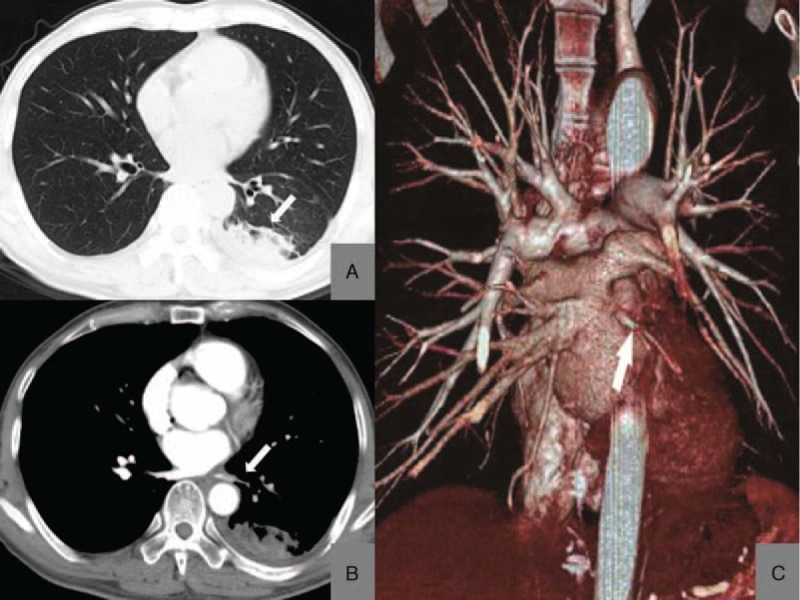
Multislice CT scan for PVS patient. (A, B) Case 3: CT shows little consolidation shadows and pleural effusion in the left lung. (C) Case 3: 3D CT angiogram shows of left inferior pulmonary veins. CT = computed tomography, PVS = pulmonary vein stenosis.
